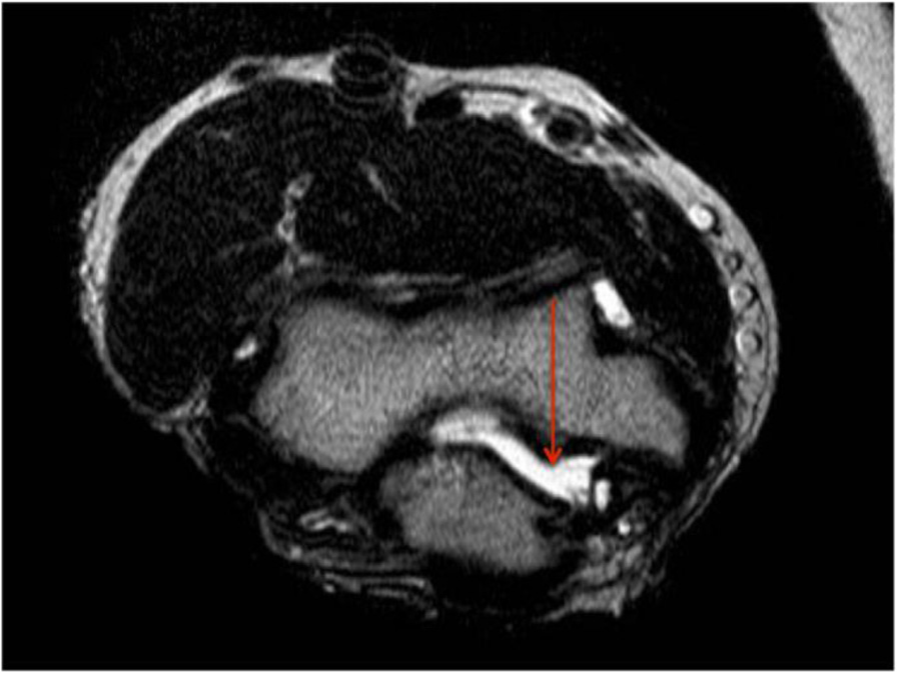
Complete tear of the MCL with humero-ulnar joint incongruence at the medial side (*red arrow*) in axial view in MRI
